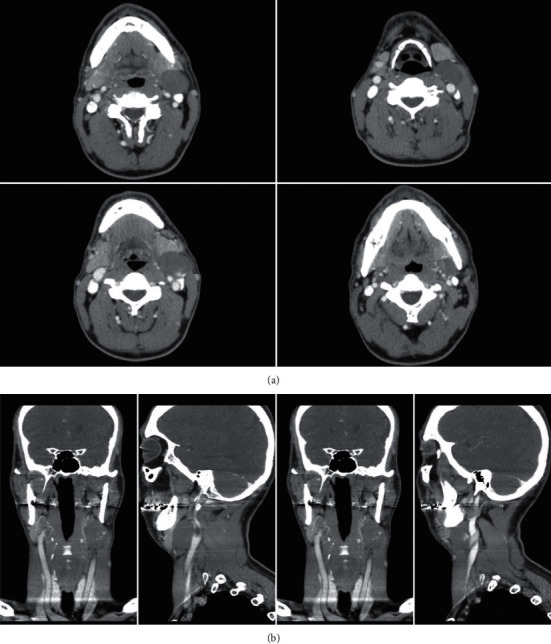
(a, b) CT scan of the head and neck revealing mass encompassing the inner and outer carotid arteries at the level of the bifurcation.
